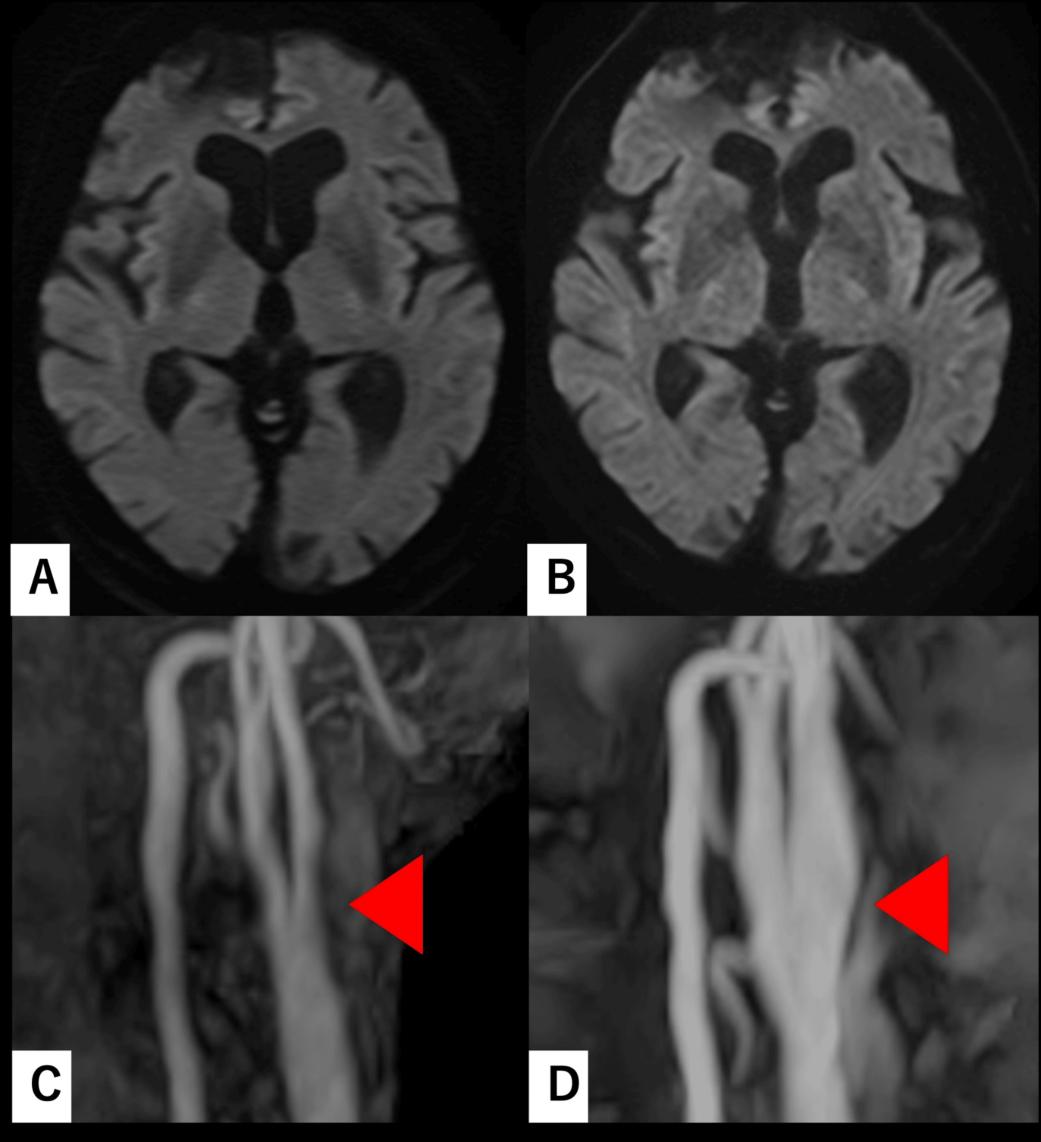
DWIs and cervical MRA images for Case 2 before and after CEA Images (A and B) show DWI scans, while images (C and D) display cervical MRA. Images (A and C) were acquired prior to CEA, and images (B and D) were obtained the day after CEA. No ischemic lesions are observed following the procedure. An increased diameter of the left ICA (arrowhead) and successful vascular reconstruction are evident. DWI: diffusion-weighted imaging; MRA: magnetic resonance angiography; CEA: carotid endarterectomy; ICA: internal carotid artery
